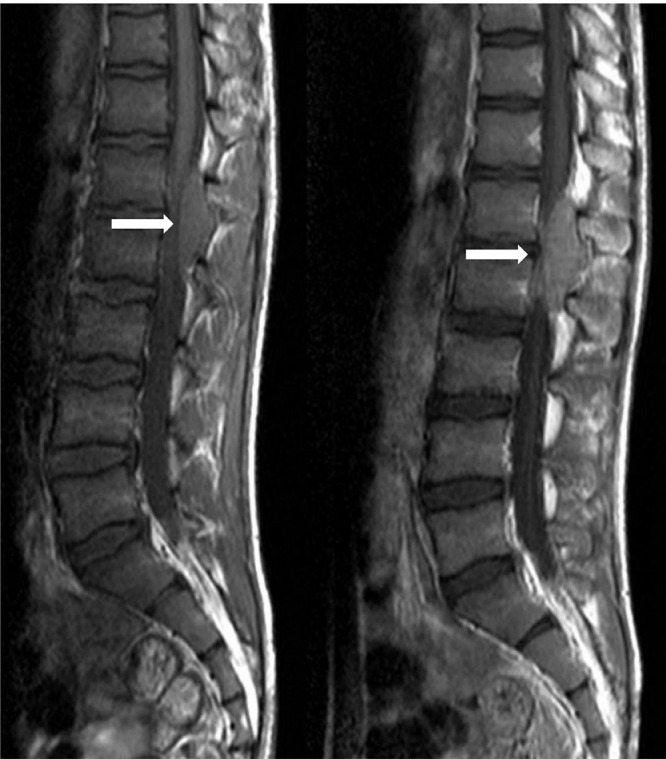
lumbosacral magnetic resonance imaging (MRI) without and with gadolinium revealed an intradural extramedullary mass in the spinal canal at L1-L2 level. White arrows indicate mass lesion.
